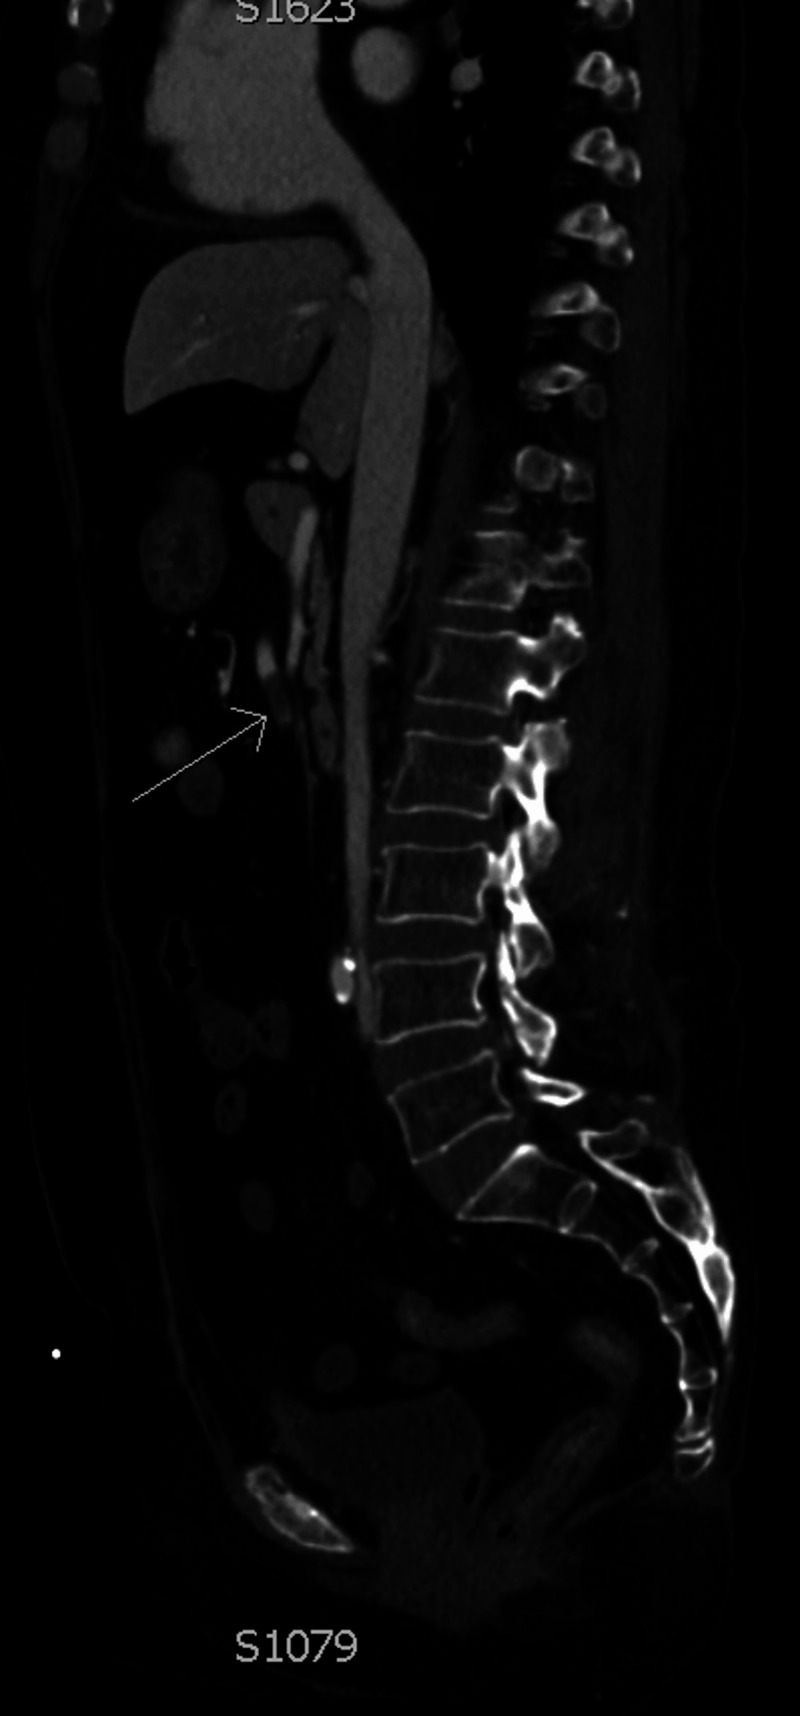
Sagittal CT angiography demonstrating the superior mesenteric artery with thromboembolism (arrow) and lack of distal flow
